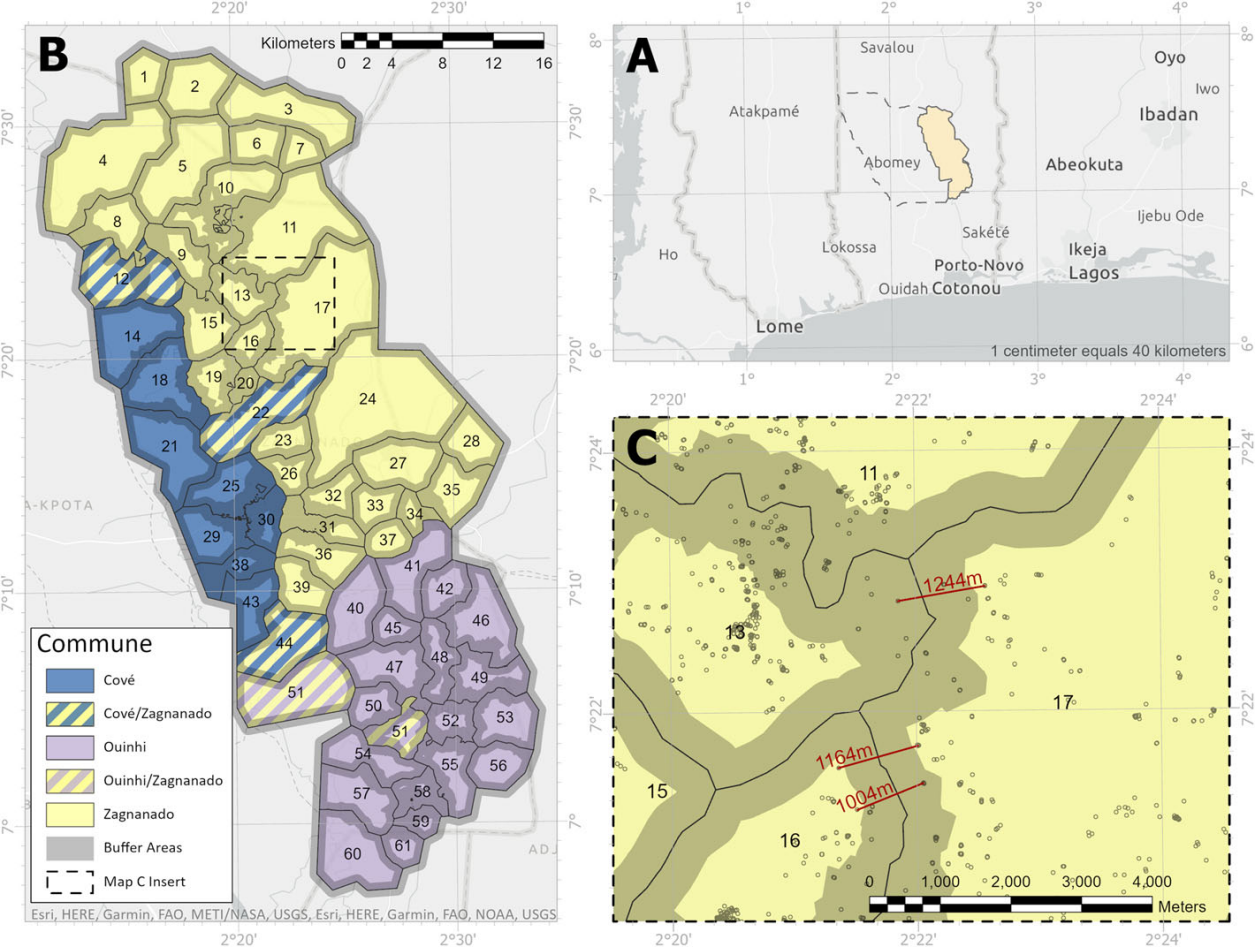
Study area. Map showing Cove, Zagnanado and Ouinhi Districts, located in Zou department, central Benin, West Africa (Panel a); the 60 study clusters identified with core and buffer area and intervention allocation (Panel b); a minimum of 1000 m area was created between households in adjacent clusters (Panel c). Source of map: Own from the study investigators (CT, JC, MA, ED).
